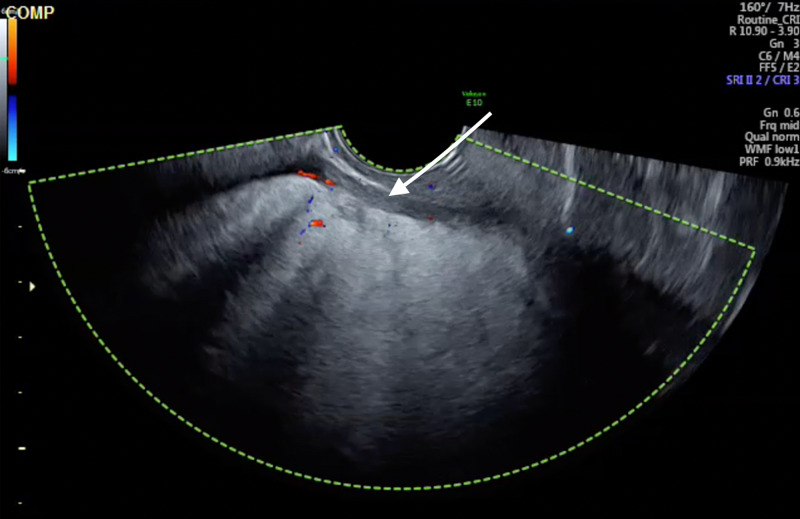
Transvaginal pelvic ultrasound image with colour Doppler revealing a markedly echogenic midline pelvic mass with minimal internal vascularity (white arrow).
